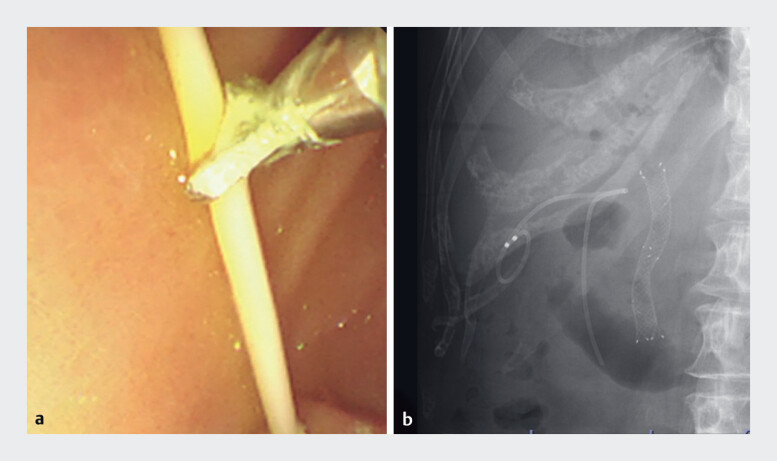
Endoscopic internalization by cutting a transmural naso-gallbladder drainage tube under endoscopic ultrasound guidance.
